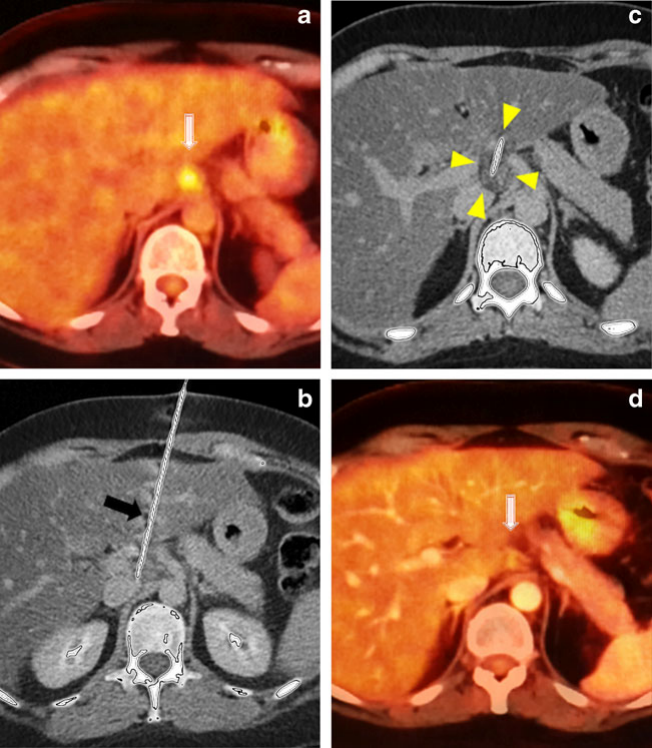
Cryoablation of a peripancreatic lymph node. a In a 44-year-old patient after surgical treatment of ovarian carcinoma, PET-CT image shows a lymph node metastasis (*white arrow*) near the pancreas. Since the metastasis was solitary, percutaneous ablation was planned, and because of the critical location of the lesion, cryoablation was preferred. b Under local anaesthesia and US+CT guidance, a cryoablation needle (*black arrow*) was inserted transhepatically into the lesion. c The lymph node was ablated with two freeze and one thaw cycle, and an iceball was typically seen (*arrowheads*). Since the liver was traversed, track ablation was performed after cryoablation to prevent bleeding and tumour seeding. d Eight months later, the follow-up CT shows complete metabolic response of the lesion (*white arrow*)
